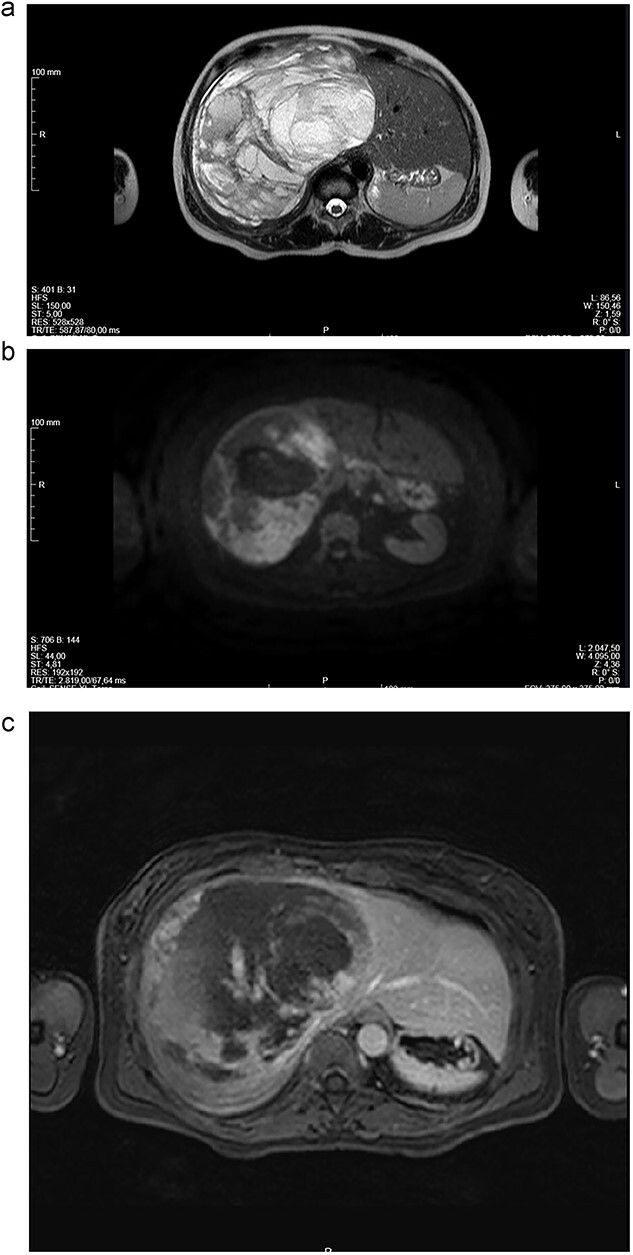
(a-c) Magnetic resonance imaging (MRI) scans of a woman aged 49 years with undifferentiated embryonal sarcoma of the liver. Liver of increased dimensions, due to the presence of a voluminous oval mass and well-defined limits occupying the entire right lobe. Heterogeneous mass with enhanced thin septa in the right lobe of the liver with fluid levels suggestive of atypical hemangioma. The right hepatic vein not dissociable to the tumor.
